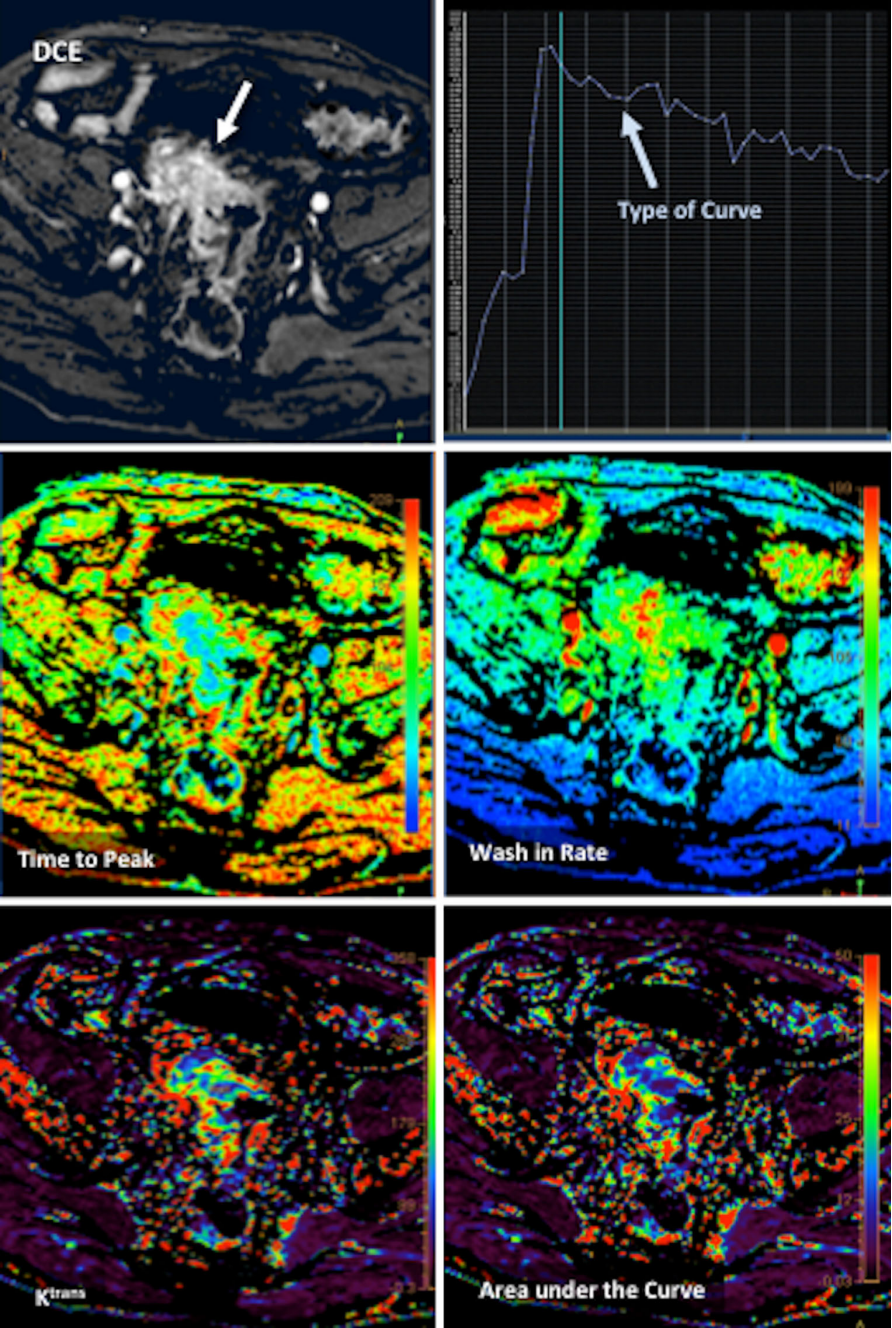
Dynamic contrast-enhanced (DCE) MRI evaluation of a rectal malignant tumour (white arrow) with different types of parameters. Data analysis of DCE-MRI data may be based on different approaches. A qualitative evaluation is based on the visual assessment of tumour enhancement (top left) or type of curve enhancement (top right). Semiquantitative parameters can characterize the shape and structure of the curves of enhancement (middle row). Finally, a quantitative approach is able to measure physiological parameters (bottom)
